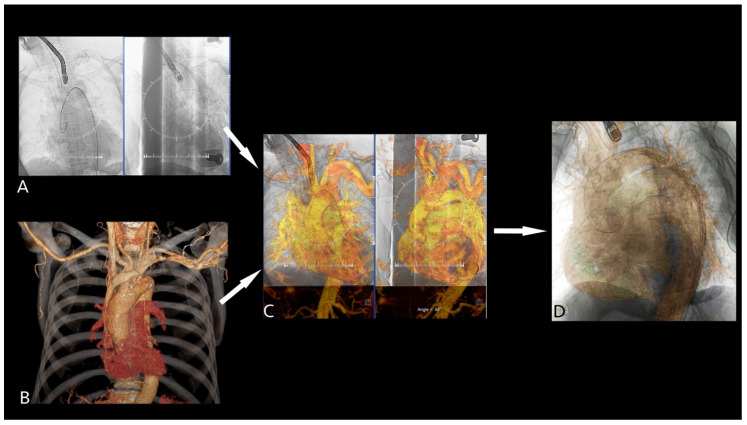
Following stiff wire introduction to the aortic arch, fluoroscopy was acquired in anterior and LAO 60 view (A). The preoperative CTA (B) was used for fusion (C). The anatomically relevant structures were annotated, and the mask was overlayed on the live fluoroscopy image (D). Abbreviations: CTA–computed tomography, LAO–left anterior oblique.
